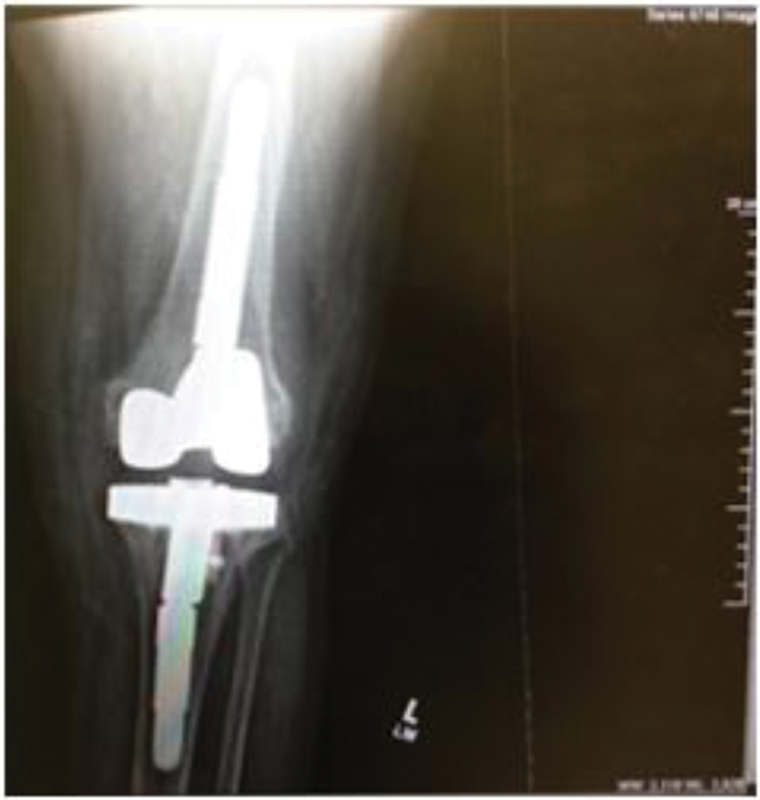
X-ray: lateral view of the insertion of a spacer postsurgery of the left knee.
